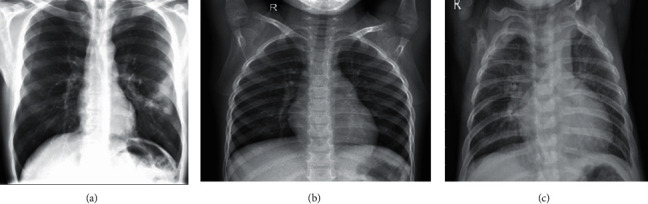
Chest X-ray data [30]: (a) COVID-19 positive, (b) normal healthy lung, and (c) regular pneumonia.
